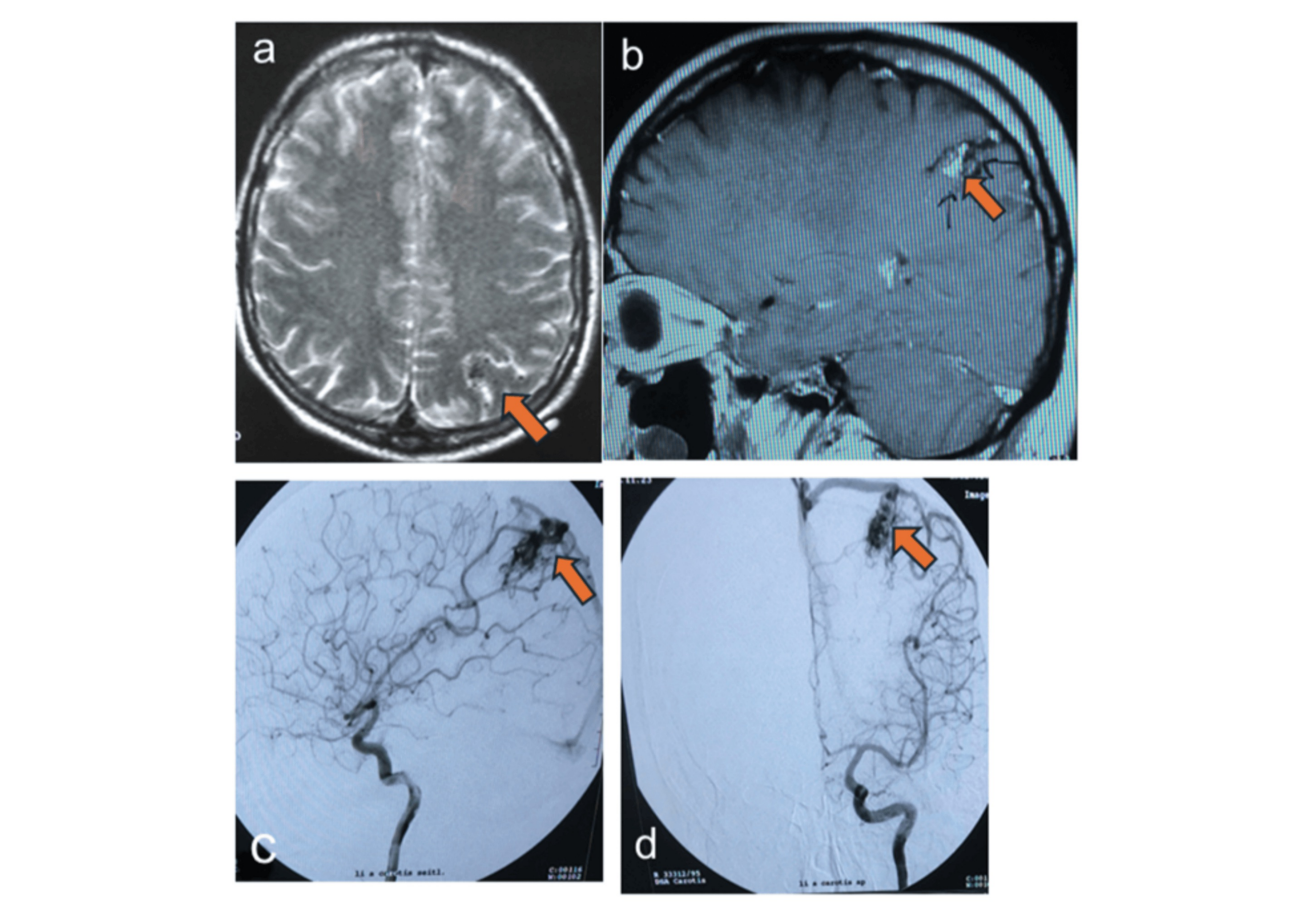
Left parietal arteriovenous malformation. (a) Axial T2-weighted MRI showing subarachnoidal flow voids left parietal (red arrow), indicating vascular structures. There is no parenchymal edema. (b) Post-contrast sagittal T1-weighted MRI showing left parietal contrast-enhancing curvilinear structures analogous to arteriovenous malformation (red arrow). (c, d) Digital subtraction angiography of the left internal carotid artery, lateral (c) and anteroposterior (d) views, showing an arteriovenous malformation with a nidus supplied by the anterior and posterior parietal branches of the inferior ramus of the left middle cerebral artery and the parietal branch of the callosomarginal artery from the left anterior cerebral artery.
